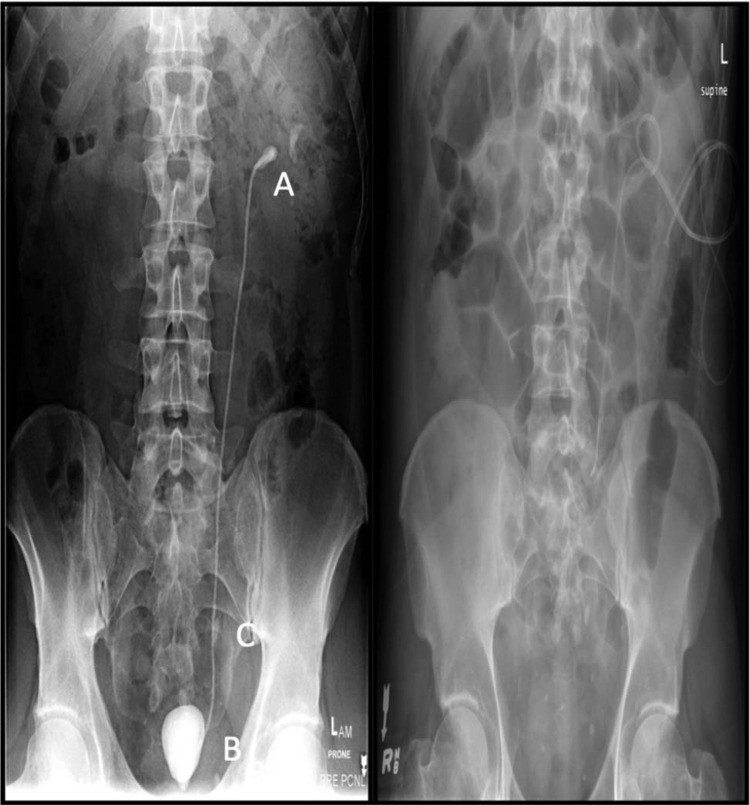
Degree of stone burden associated with 12 years with the indwelling stent. Plain film KUB radiograph shows the proximal and distal calcifications associated with the Double-J stent (A, B), respectively. The original ureteral stone is seen at the level of S2–S3 (C). The patient underwent a combination of left percutaneous nephrolithotomy and cystolitholapaxy to remove the stent and its associated stones. The original left mid-ureteral stone was subsequently treated with URS and laser lithotripsy, after failed shockwave lithotripsy treatment. The patient was left with a stent with a dangle string after the final URS, which was finally removed in outpatient follow–up, leaving him stone and stent free. He remains stone free on follow-up.
